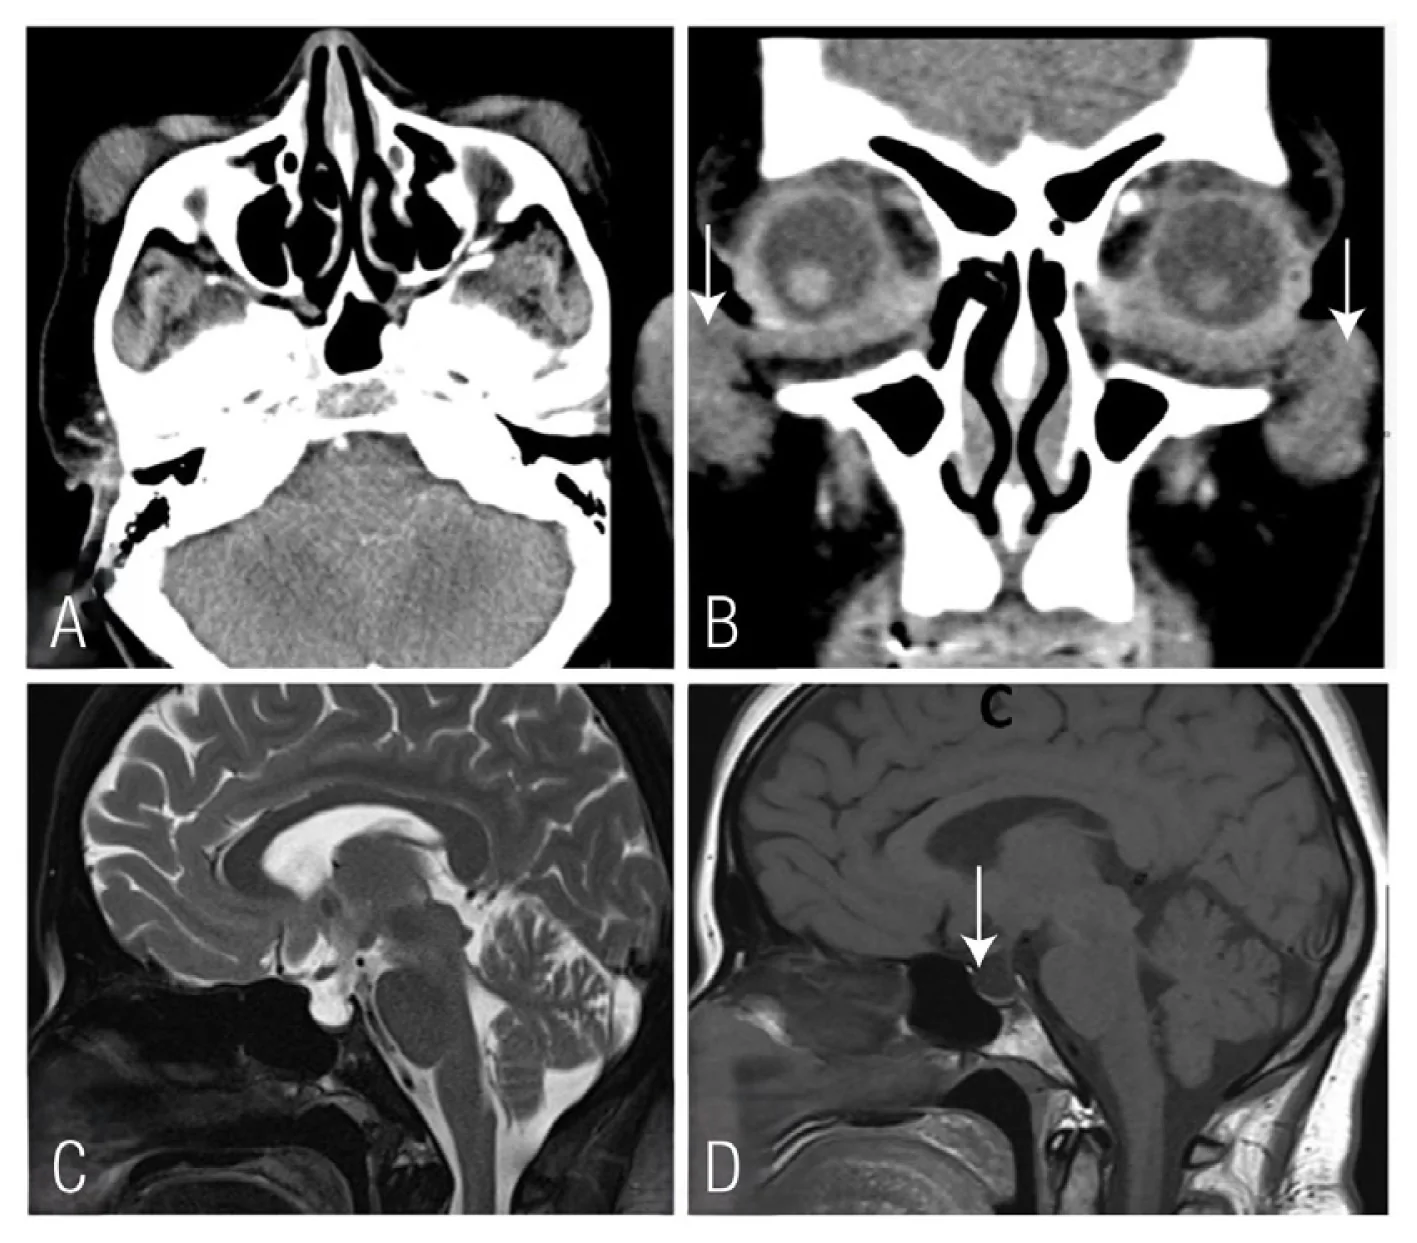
Computed tomography of the (A) axial and (B) coronal reformation showing soft tissue density mass lesions in prezygomatic areas bilaterally with no calcification or underlying bony involvement. Magnetic resonance imaging of the brain in sagittal (C) T2 and (D) T1 revealing non-visualisation of the pituitary gland with pituitary compressed along the sellar floor with pituitary fossa filled with cerebrospinal fluid signals and pituitary stalk reaching up to the sellar floor. Features are of empty sella syndrome.
